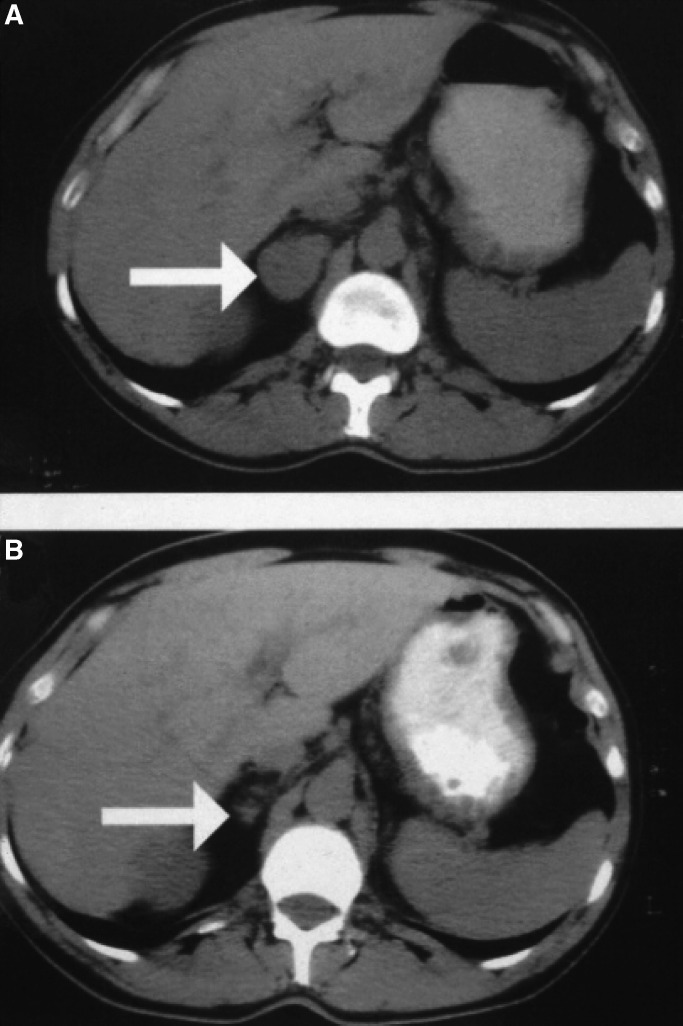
Response to treatment in a patient with renal carcinoma. CT sections imaging the right adrenal gland (arrowed) in a patient with renal carcinoma. (A) Baseline scan, (B) scan after 16 weeks of treatment.
